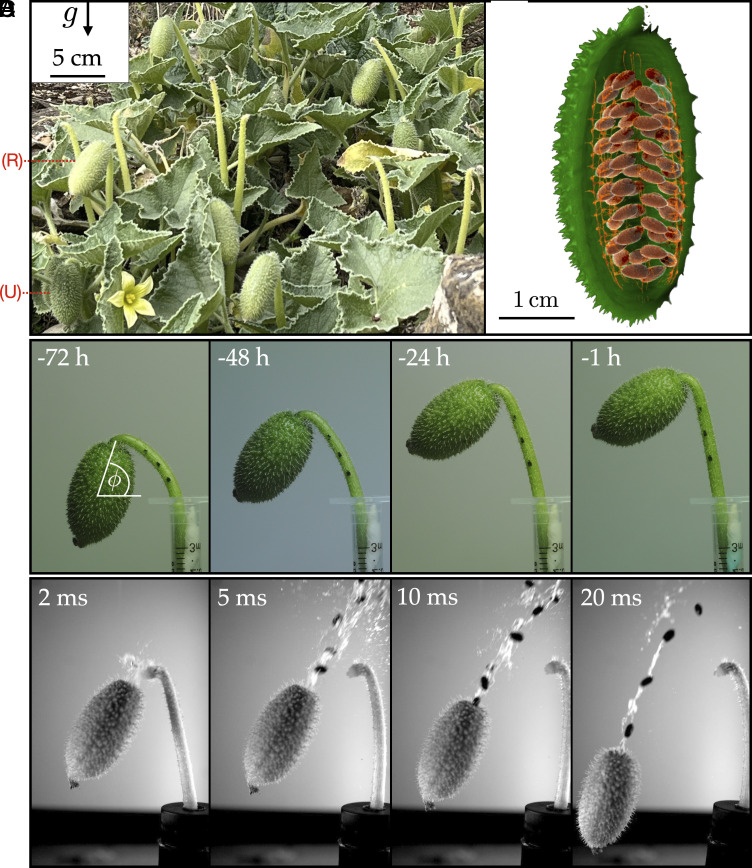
The squirting cucumber. (*A*) Images of *Ecballium elaterium* in the Oxford Botanic Garden. (R and U) indicate ripe and unripe fruits. (*B*) Computed Tomography (CT) scan of the cucumber shows how the seeds are arranged in pairs attached to four pillars located at 90 degree intervals around the long axis of the fruit. (For CT scan video see Movie S1.) (*C*) Images of fruit reorientation at t-minus 72, 48, 24, and 1 h (*Left*-to-*Right*) to launch. (*D*) Images of seed dispersal at 2, 5, 10, and 20 ms (*Left*-to-*Right*) after abscission of the stem.
